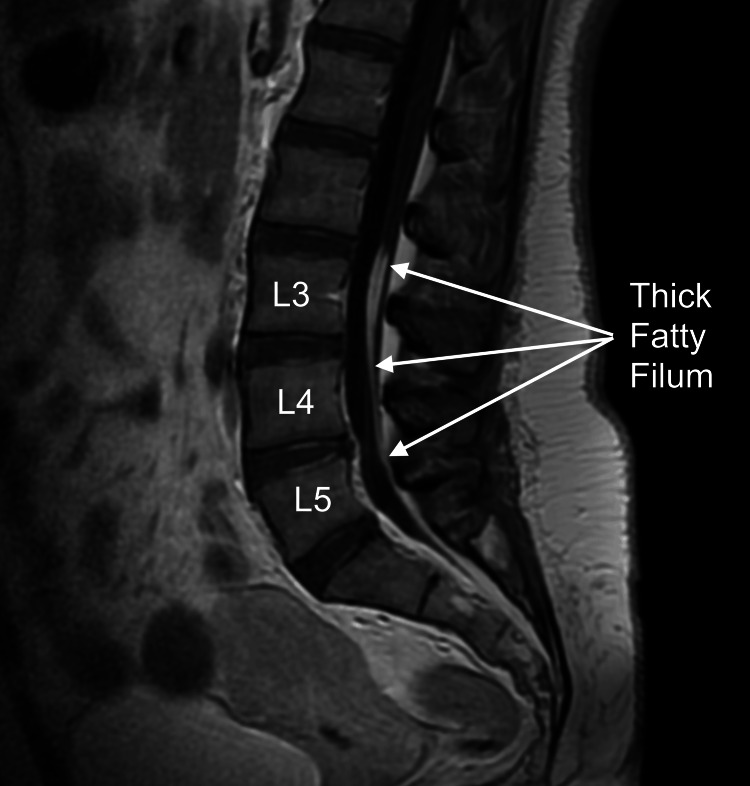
Lumbar magnetic resonance imaging. Sagittal T1-weighted image. Fatty filum extending from L3 to L5, with a maximum diameter of 5.5 mm at L3.
